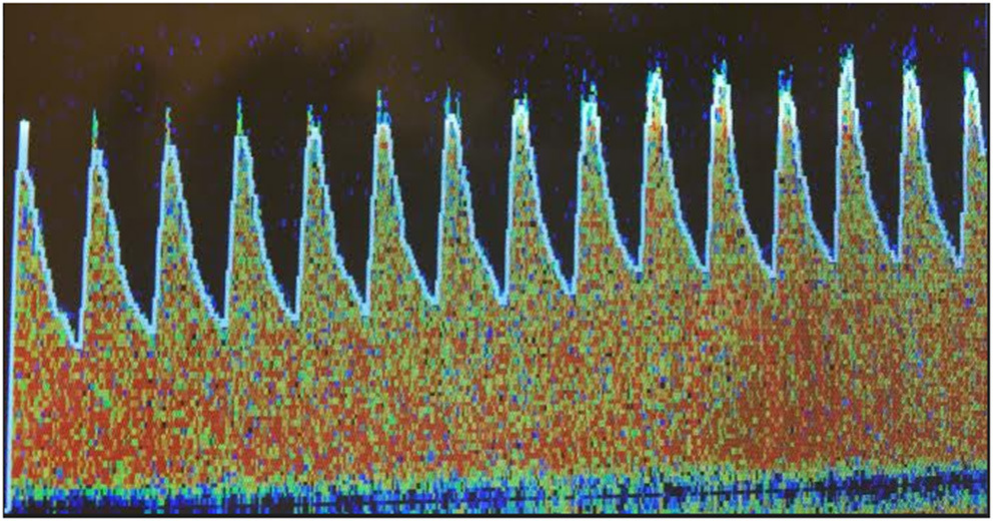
Example of transcranial Doppler ultrasound of middle cerebral artery showing increased mean flow velocity during breath-hold test as a sign of preserved cerebrovascular reserve capacity.
